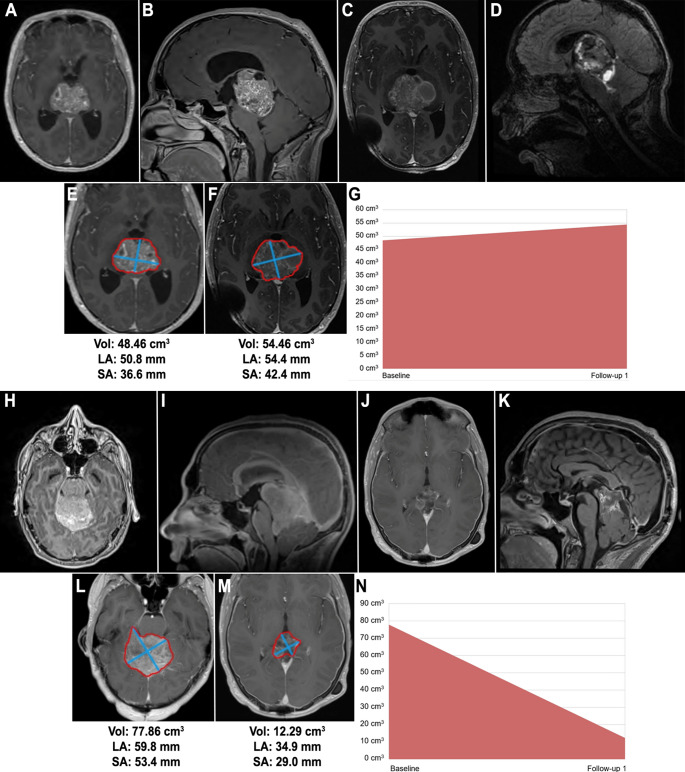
Case 1. Imaging of the only patient in our series whose tumor progressed on chemotherapy. This was a 16-year-old male with a PAG. Pre-chemotherapy (A) axial, and (B) sagittal T1-weighted with contrast MRI demonstrating the pineal mass. Post-chemotherapy (C) axial, and (D) sagittal T1-weighted with contrast MRI imaging demonstrating an increase in the volume of the mass. MRI tumor analytics in (E) and (F) show the calculated volume (cm^3^), long axis (mm), and short axis (mm) of the mass. (G) Visual depiction of an increase in tumor volume by 12%. Case 2. Imaging of a 22-year-old male with a pineoblastoma that decreased in size during pre-operative chemotherapy. Pre-chemotherapy (H) axial, and (I) sagittal T1-weighted with contrast MRI demonstrating a pineal mass. Post-chemotherapy (J) axial, and (K) sagittal T1-weighted with contrast MRI imaging demonstrating a decrease in the volume of the mass. MRI tumor analytics in (L) and (M) showing the calculated volume (cm^3^), long axis (mm), and short axis (mm) of the mass. (N) Visual depiction of a decrease in tumor volume by 65 cm^3^ (84%), the maximum absolute tumor volume reduction in our series
